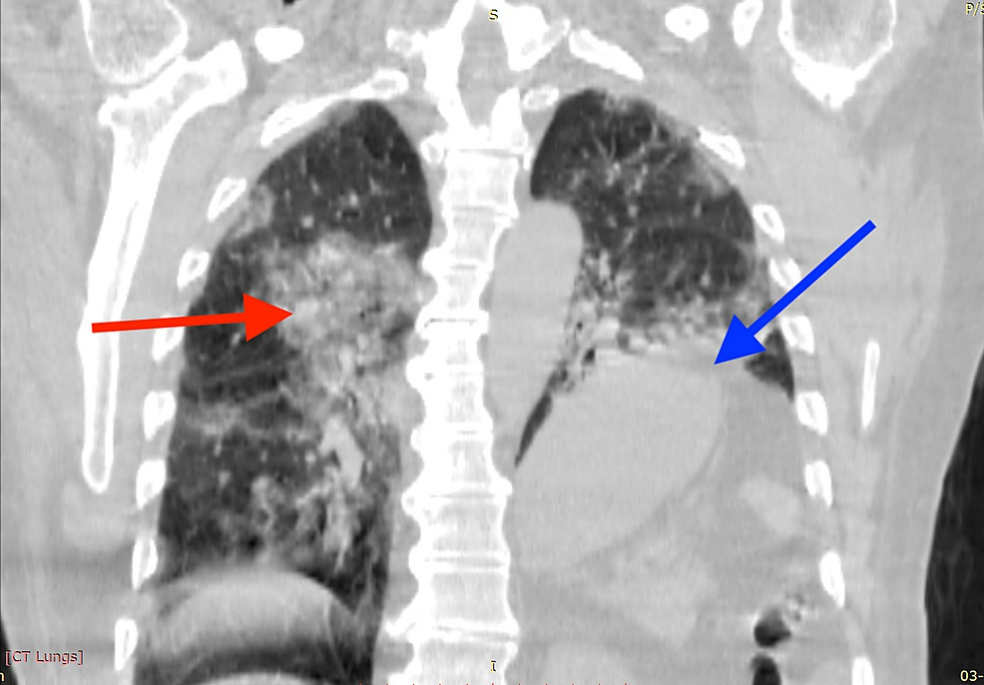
Coronal section chest HRCT showing bilateral multifocal peripheral ground glass opacities (red arrow) with a raised left hemidiaphragm (blue arrow)
